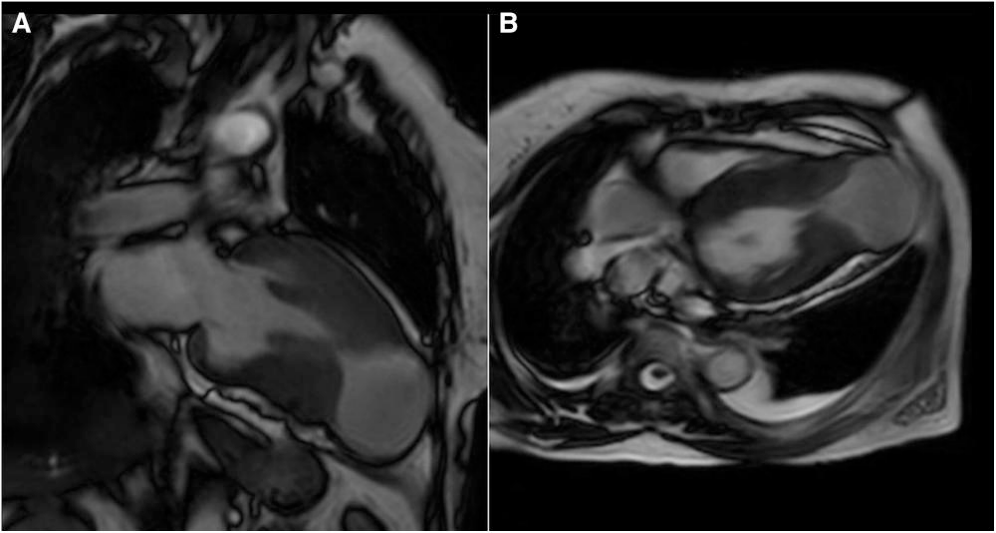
Cardiac MRI demonstrating the apical aneurysm and apical and mid-septal hypertrophy in *(A)* two-chamber and *(B)* four-chamber long-axis views.
